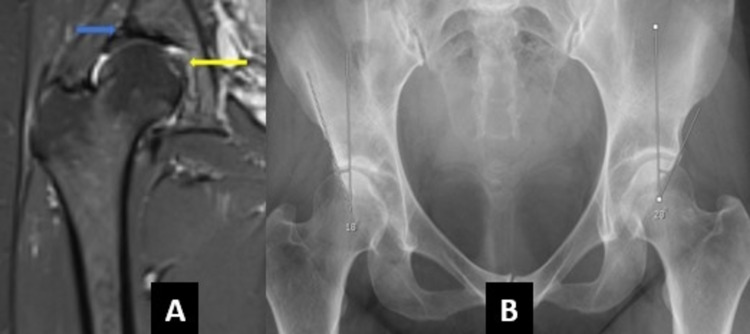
A 46-year-old patient who presented with right hip pain. (A) Coronal STIR sequence of MRI demonstrating ligamentum teres edema (yellow arrow) with the hypertrophy of the labrum (blue arrow). (B) AP pelvis view showing decreased center edge angle (18 degrees) on the right side. STIR: short tau inversion recovery; MRI: magnetic resonance imaging
